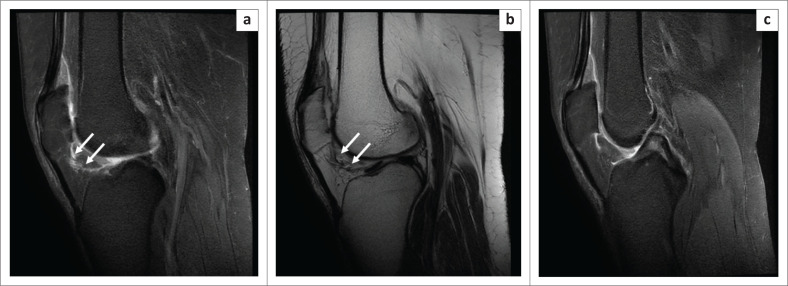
Magnetic resonance imaging of an infrapatellar plica tear in a 53-year-old female with a history of anterior knee pain: oblique sagittal PDFS image (a) and T2WI (b) reveal a hyperintense fluid collection along the entire course of the infrapatellar plica (arrows), with ragged margins. Oblique sagittal PDFS image (c) indicates a normal anterior cruciate ligament and posterior cruciate ligament in the patient.
